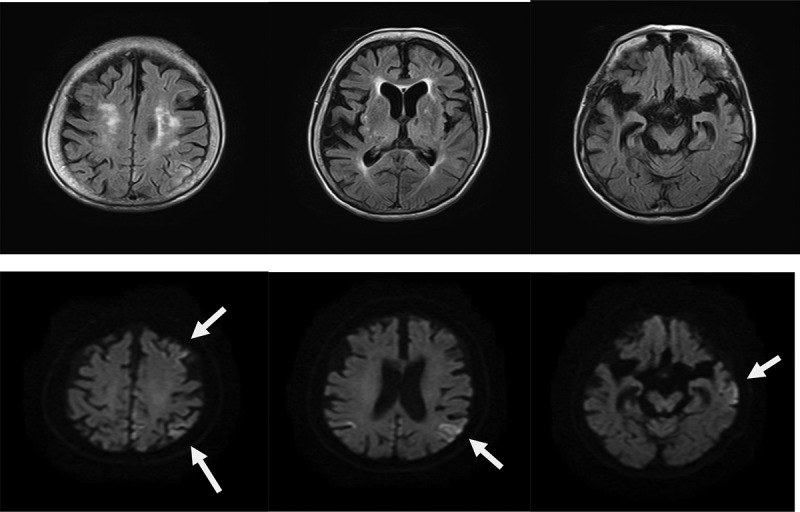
a. Magnetic resonance imaging (MRI) findings obtained using fluid-attenuated inversion-recovery (FLAIR) (upper) and diffusion-weighted images (DWI) (lower) in an 89-year-old woman. DWI shows cortical hyperintensities in some areas of both hemispheres; the white arrows indicate areas of cortical hyperintensity in the left hemisphere.
